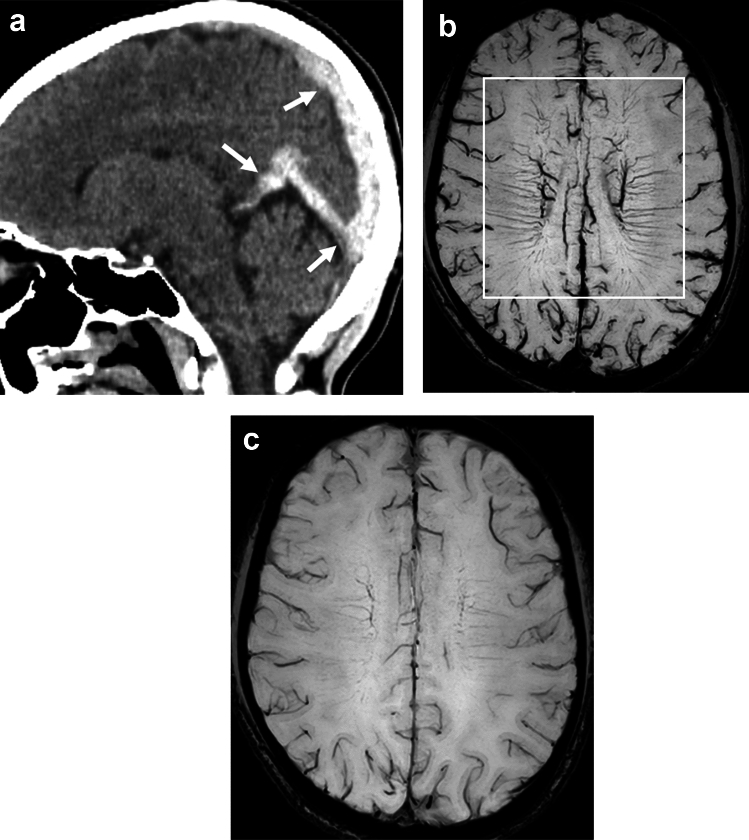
A 21-year-old woman presented with seizure. Two days after onset, CT reveals thrombi in the Galenic, straight, and superior sagittal sinuses (a, arrows). SWI shows brush sign (b, square) and prominent cortical veins bilaterally. SWI shows disappearance of the brush sign and prominent cortical veins 10 days after anticoagulation treatment (c)
